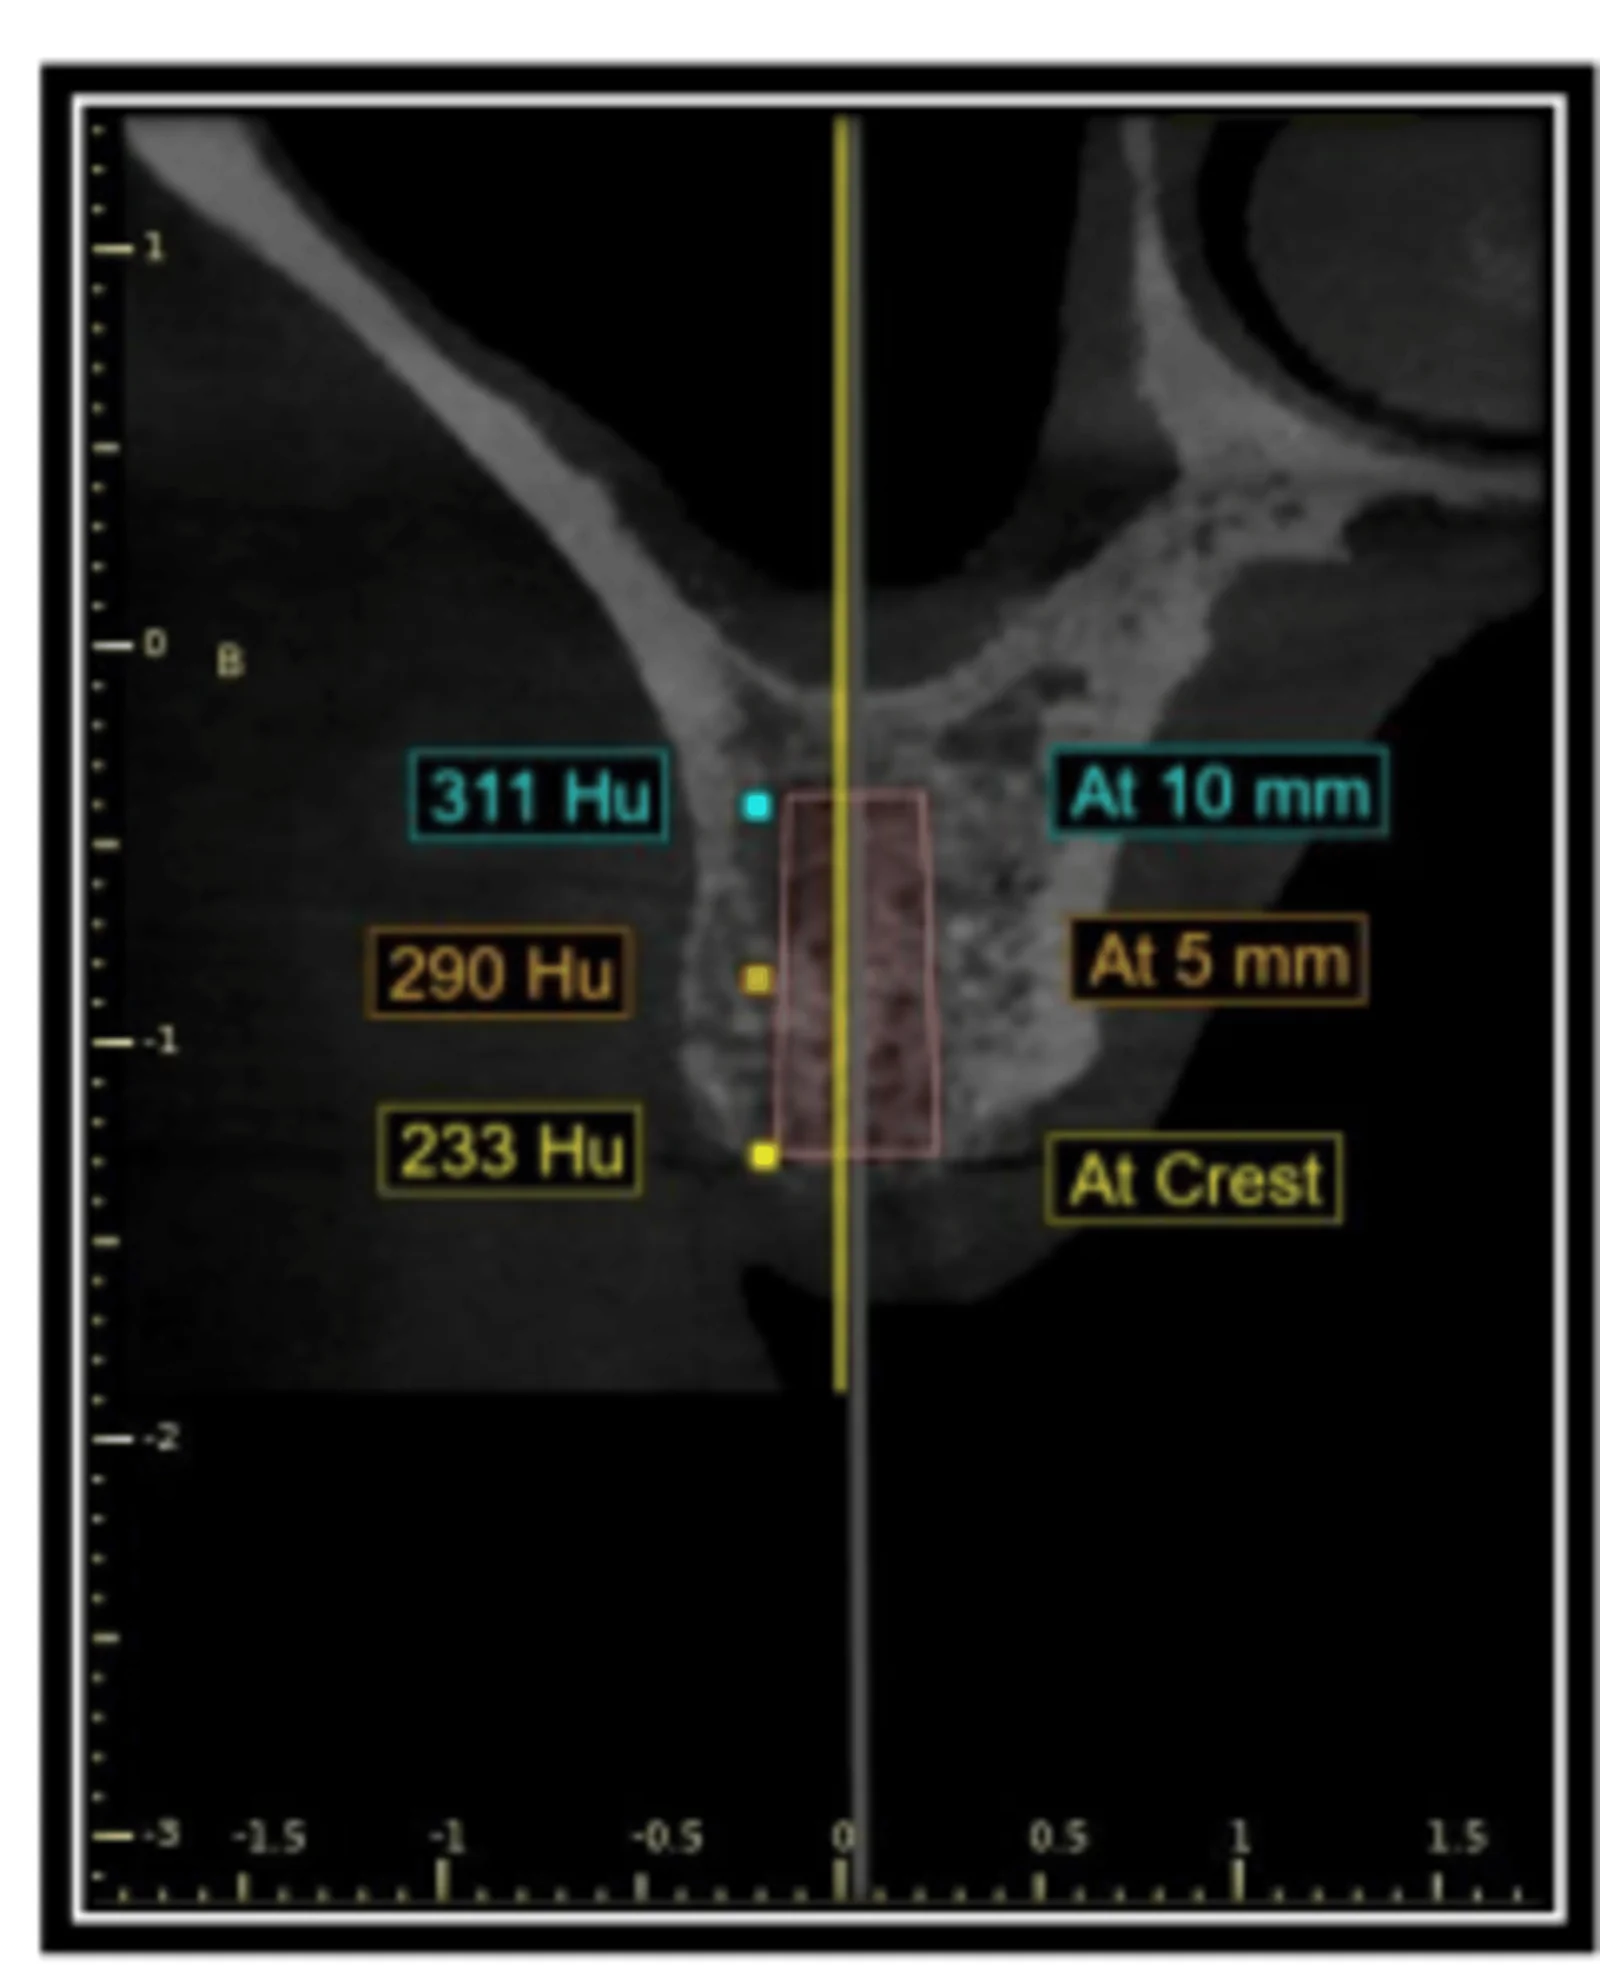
Representative cone-beam computed tomography image showing assessment of peri-implant bone density and alveolar ridge width in the posterior maxilla at L1, L2, and L3 levels. L1 = at the crestal level; L2 =  5 mm above L1; L3 = 5 mm above L2
